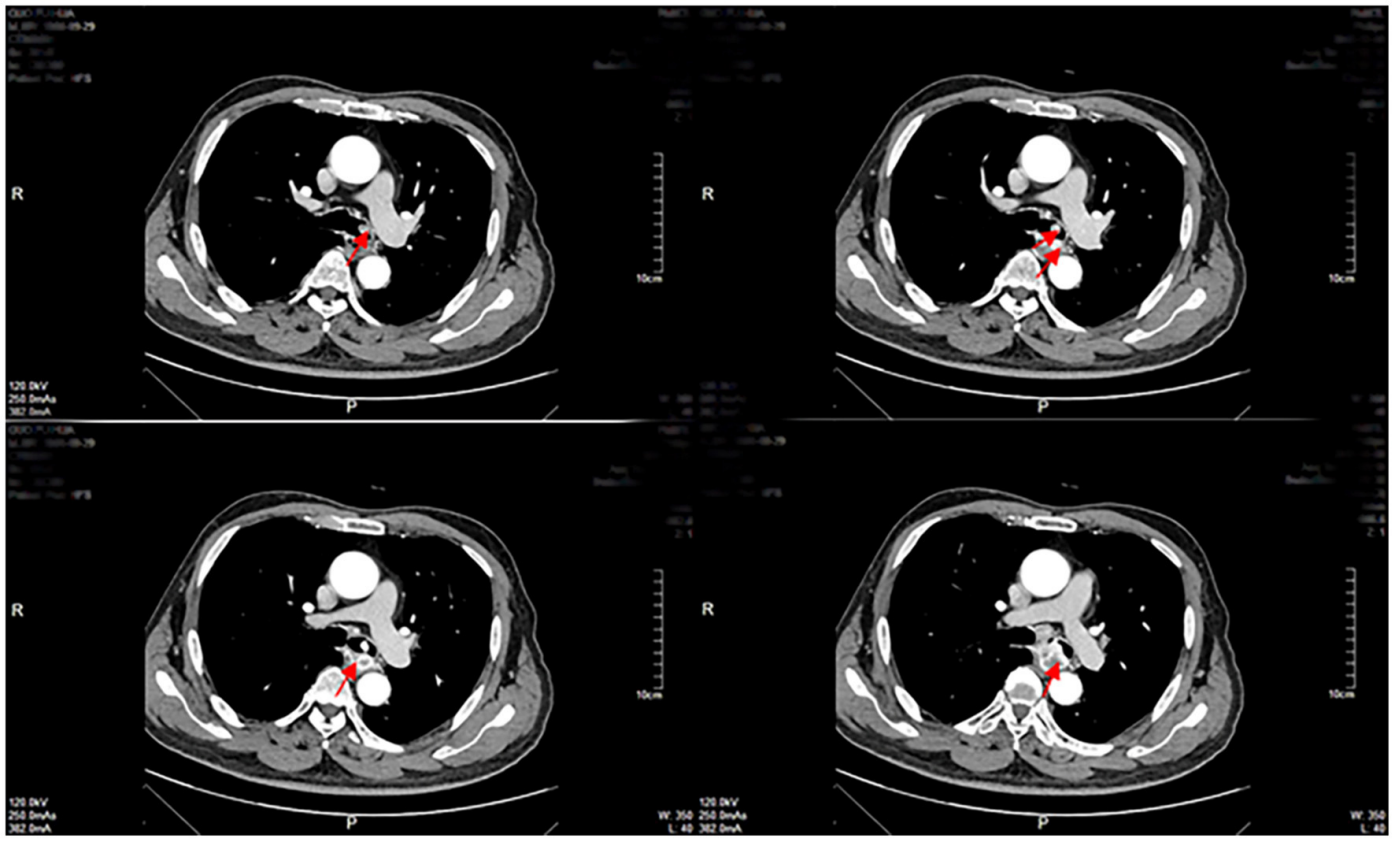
Enhanced CT examinations revealed a mass of inhomogeneous shadow located in the left main bronchus close to the carina as shown by the arrows.
